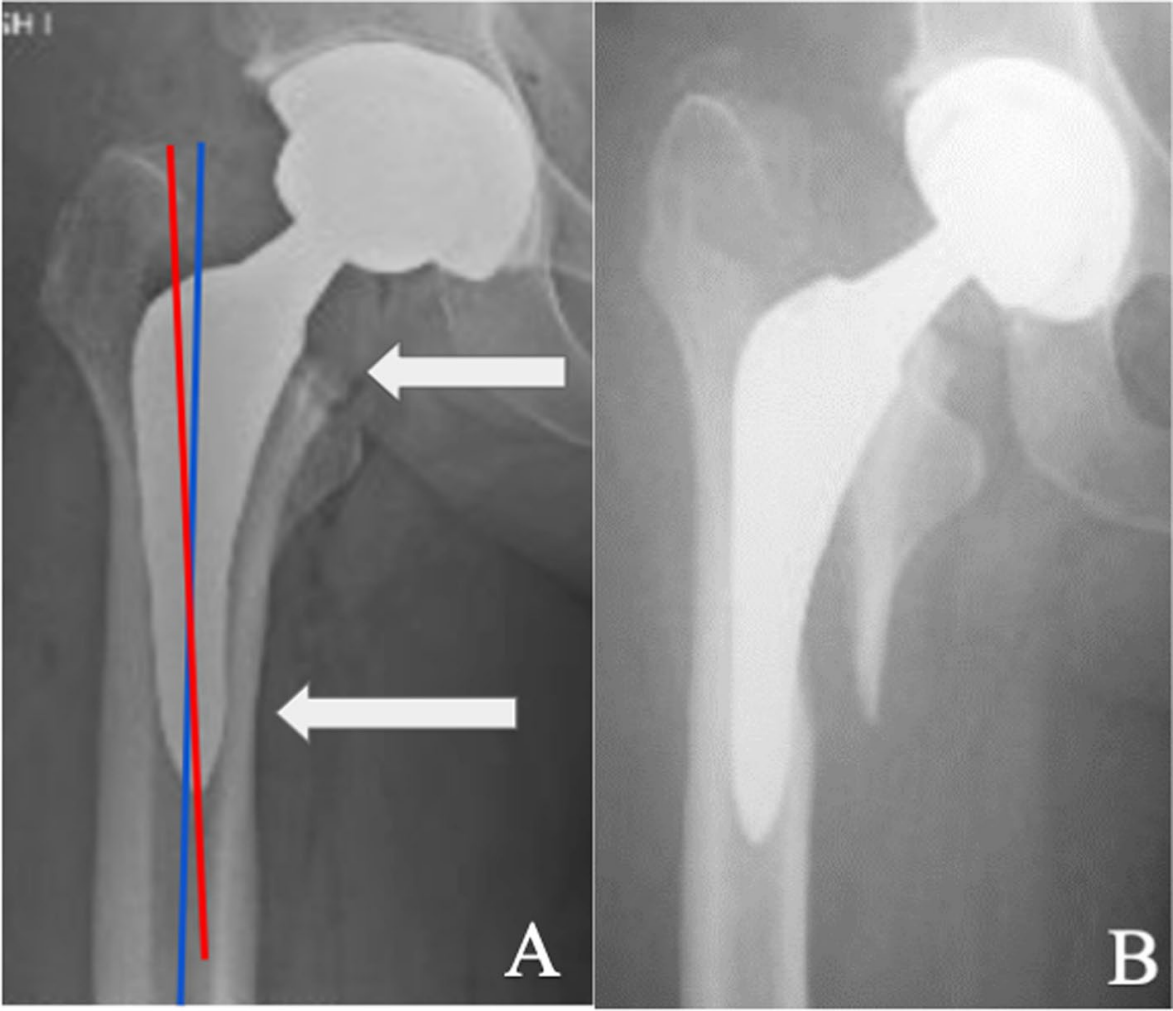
Figure A (left) demonstrates the initial postoperative radiograph with an extended offset femoral stem in slight valgus malalignment, a low femoral neck cut, and the stem positioned proud in relation to the femoral neck cut. Figure B (right) demonstrates the resulting Vancouver B2 fracture with the apex of the medial proximal femur fragment proximal to the stem tip
